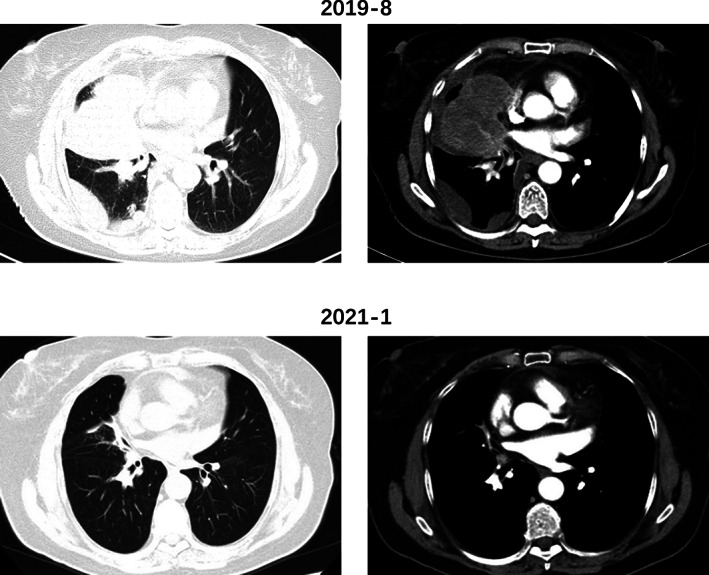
Chest CT before and after treatment with camrelizumab + apatinib
